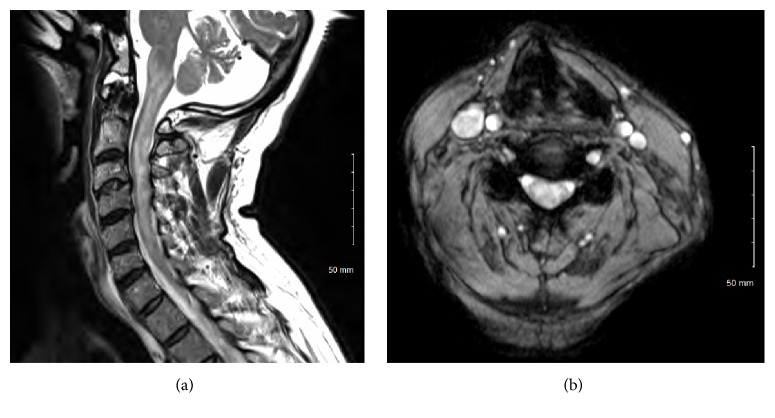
Preoperative T2-sequence MRI of the cervical spine. Sagittal (a) and axial (b) images showing diffuse spinal cord edema and T2-signal changes around the lesion.
